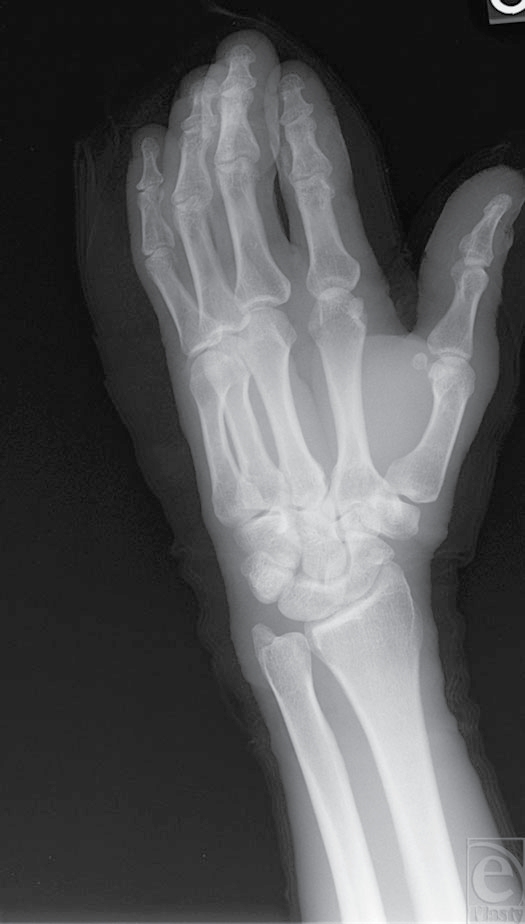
Postextraction x-ray film demonstrates no bony injury.
